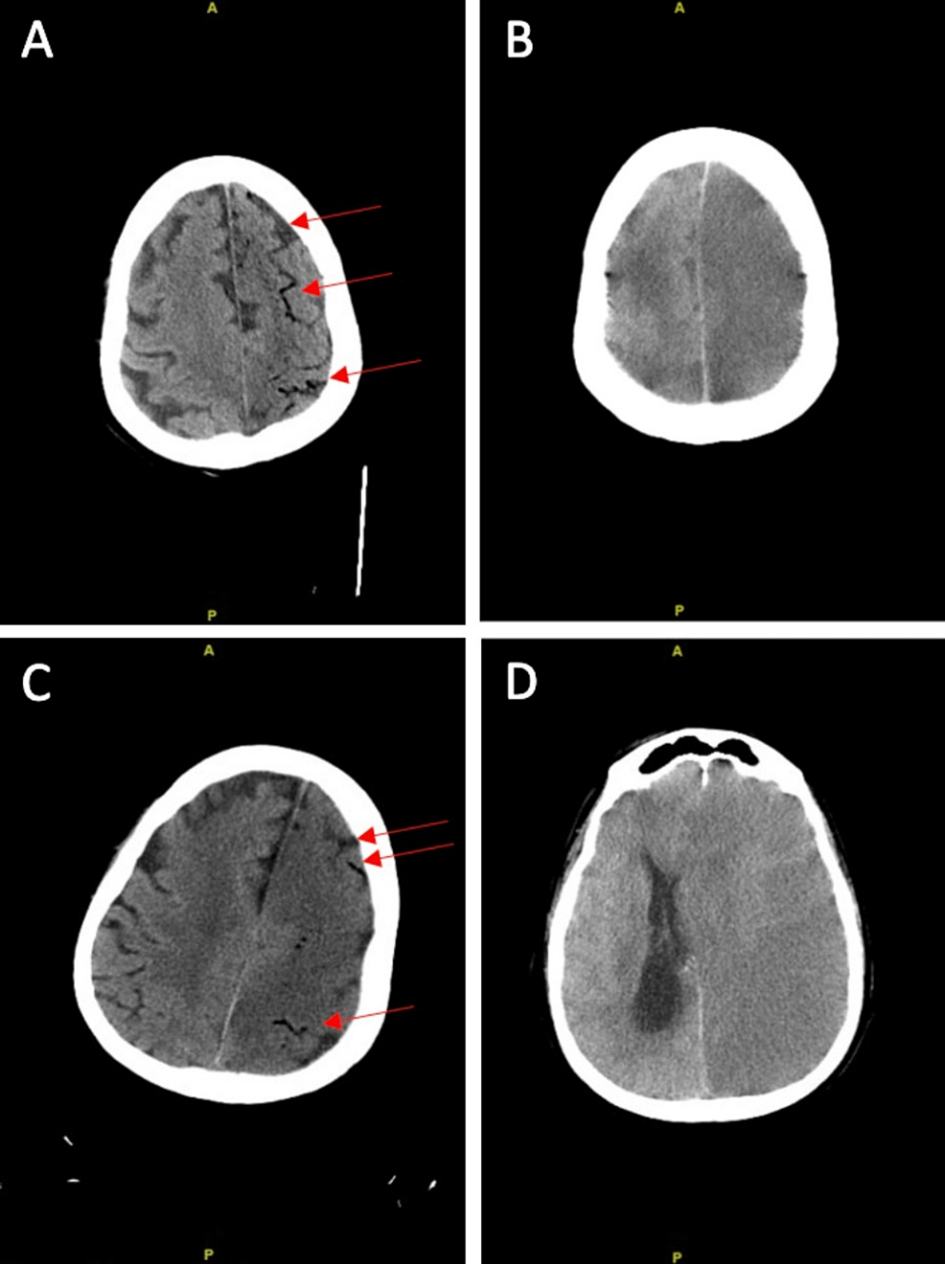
Non-contrast axial CT images of the brain over three consecutive days. (A) At initial presentation, red arrows highlighting multiple serpiginous low attenuation density foci in the frontal and parietal subarachnoid space. Differential diagnosis includes fat in the setting of ruptured dermoid versus air. (B) Twenty-four hours after initial presentation, resolution of low-density foci compatible with air dissipation, prompting diagnosis of air embolism. (C) Twenty-four hours after initial presentation, loss of normal grey-white matter differentiation involving the left hemisphere. Red arrows demonstrating persistent low-density foci compatible with air emboli. (D) Forty-eight hours after initial presentation, complete left cerebral hemisphere edema and sulcal effacement compatible with acute infarction at the level of the ventricles with associated rightward midline shift were seen.
